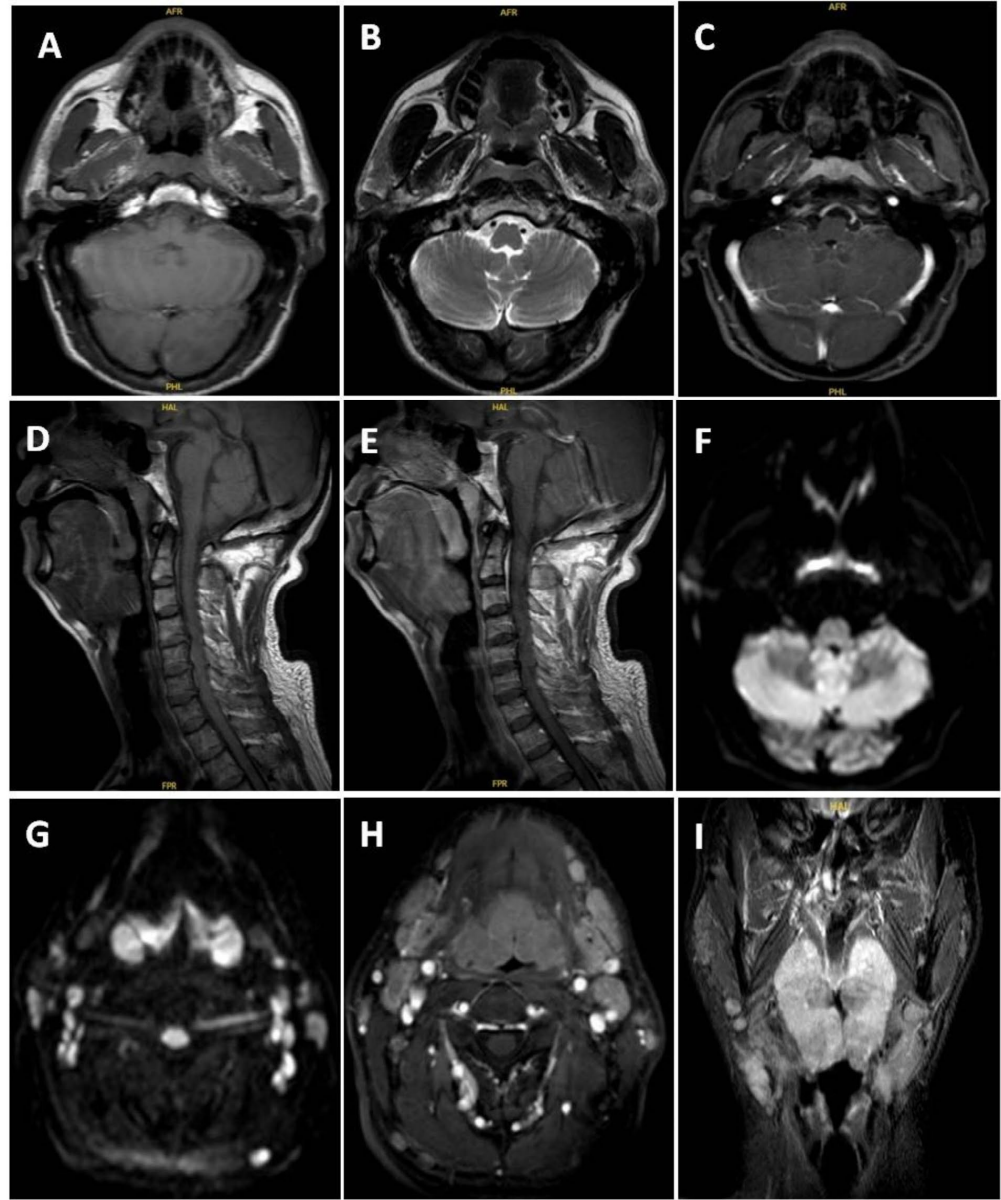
Nasopharyngeal plain and enhanced magnetic resonance imaging. A. Cross-sectional T1-weighted imaging (T1WI) showed thickening of the nasopharynx. B. Cross-sectional T2-weighted imaging (T2WI) showed thickening of the nasopharynx (the signal was uneven and exhibited boundedness, with a range of approximately 7 × 5 mm). C. Cross-sectional T1WI with contrast showed uneven and significant enhancement in the thickened area of the nasopharynx. D. Sagittal T1WI showed thickening of the nasopharynx and pharyngeal tonsils. E. Sagittal T1WI with contrast showed thickening with noticeable enhancement and enhancement of the nasopharynx and pharyngeal tonsils. F. Diffusion-weighted imaging showed limited diffusion in the nasopharyngeal lesion area. G. Diffusion-weighted imaging showed limited diffusion in the pharyngeal ring and tonsil lesions and limited diffusion in the bilateral cervical lymph nodes. H. Transverse T1WI with contrast showed significant enhancement of the pharyngeal ring, tonsil lesions, and bilateral cervical lymph nodes. I. Coronal T2WI showed enlargement of the pharyngeal ring and bilateral cervical lymph nodes
